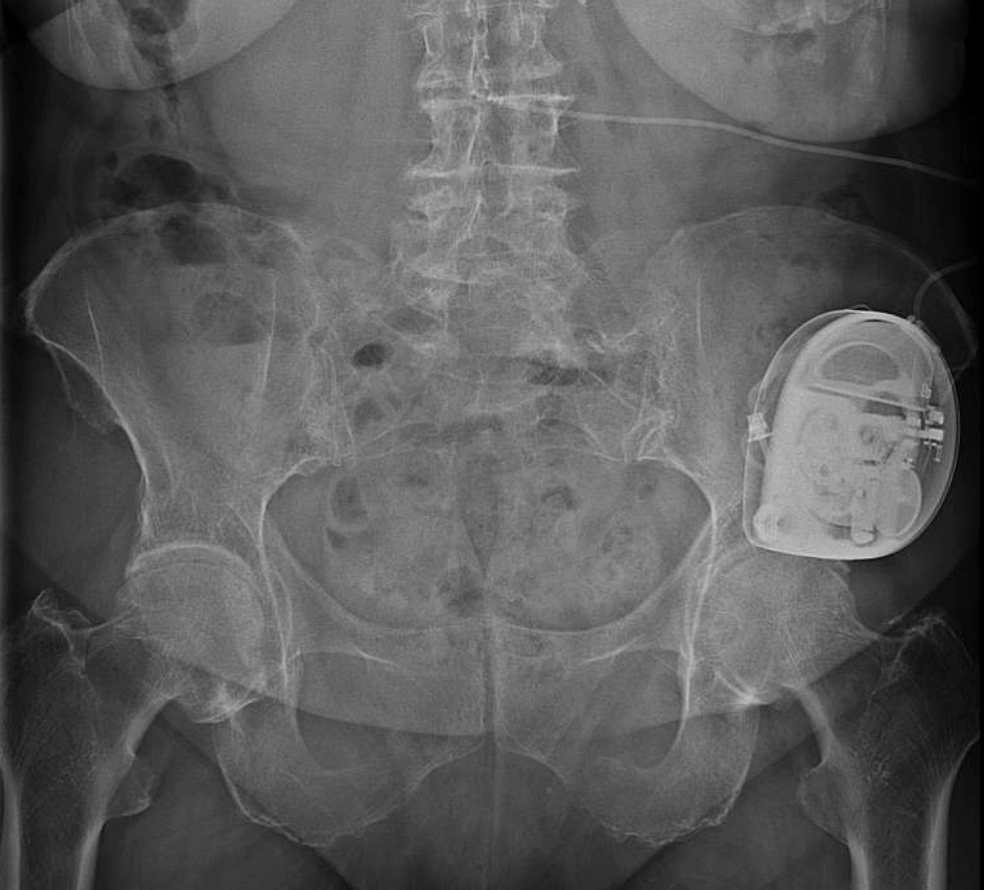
X-ray image of the pelvis depicting intrathecal pump delivering Ziconotide.
